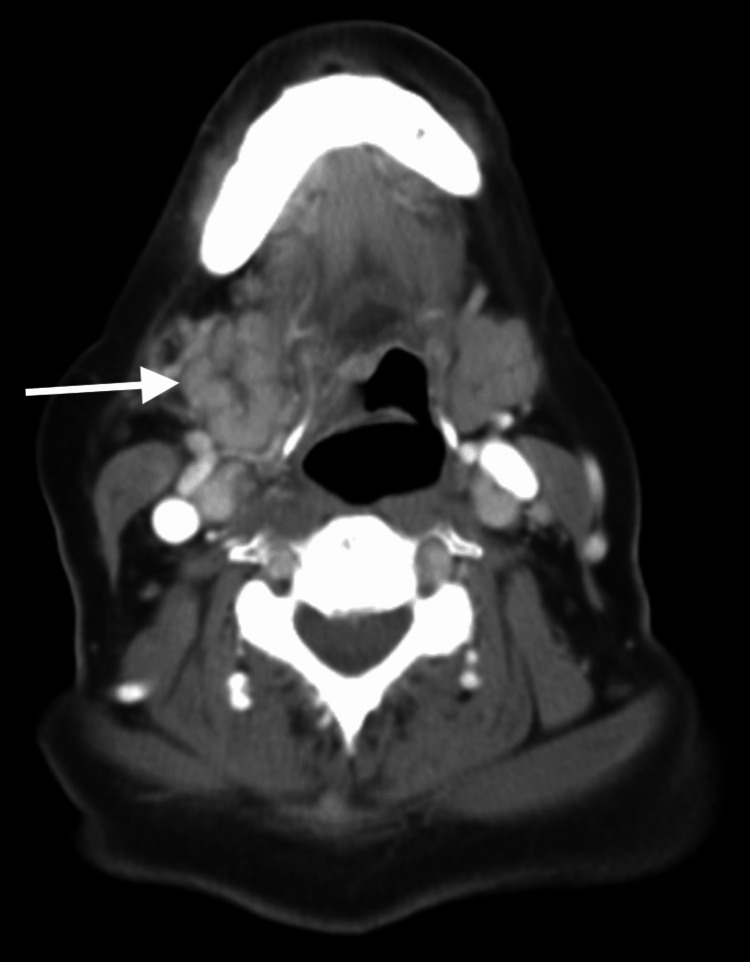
CT of the neck with IV contrast: acute sialadenitis of the right submandibular gland with mildly dilated intra and extraglandular inflamed ducts without visualization of radiopaque calculi (see arrow). CT: computed tomography; IV: intravenous
